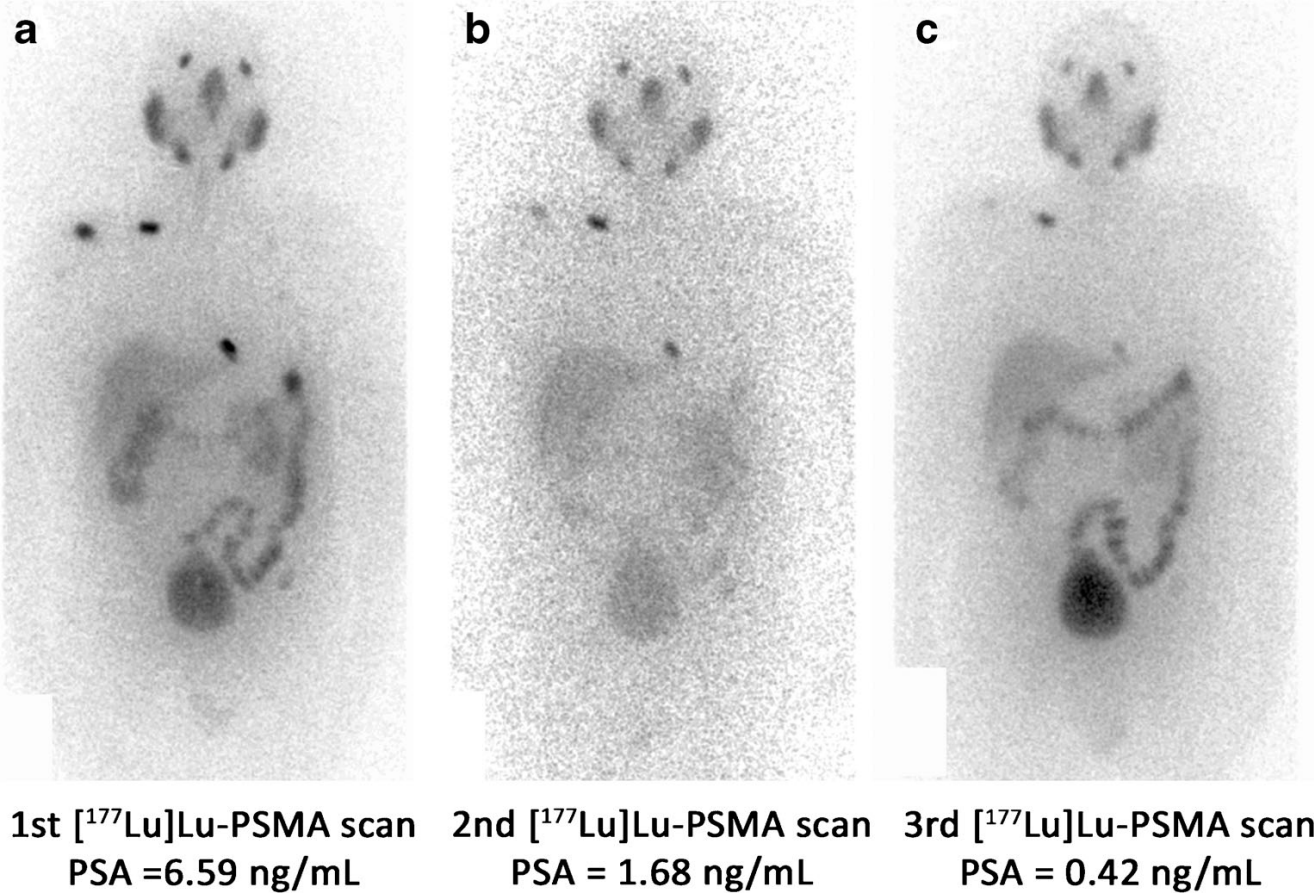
A 75-year-old male with metastatic castration-resistant prostate cancer underwent 3 cycles of Lutetium-177-prostate-specific membrane antigen ([^177^Lu]Lu-PSMA) radionuclide therapy. He had multiple bone metastases in the upper cervical vertebra, right humerus, right clavicle, both scapulae, bilateral ribs, left iliac bone, spinous process of the mid lumbar spine. The first post-treatment (PT) planar whole-body [^177^Lu]Lu-PSMA image (a) showed PSMA-avid lesions in the upper cervical vertebra, right humerus, right clavicle, a left lower rib, as well as the spinous process of the mid lumbar spine (seen in the posterior view). The subsequent PT images (b and c) demonstrated decreasing uptake in the lesions, and the prostate-specific antigen (PSA) level was declining. The PSA level was stable after 2 months of the third cycle (0.45 ng/mL). The response was favorable despite uptake in a few lesions in the post-therapy image
